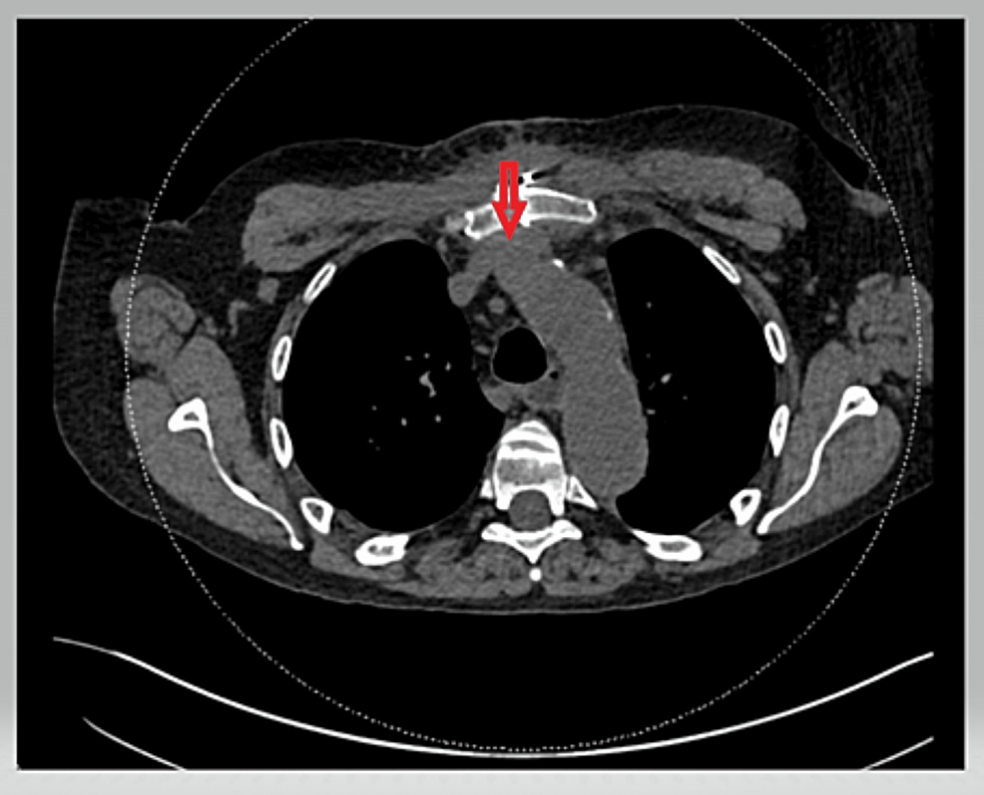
Non-contrast chest CT performed two months later showing the left brachiocephalic vein had returned to its previous caliber without stenosis. CT: computed tomography
